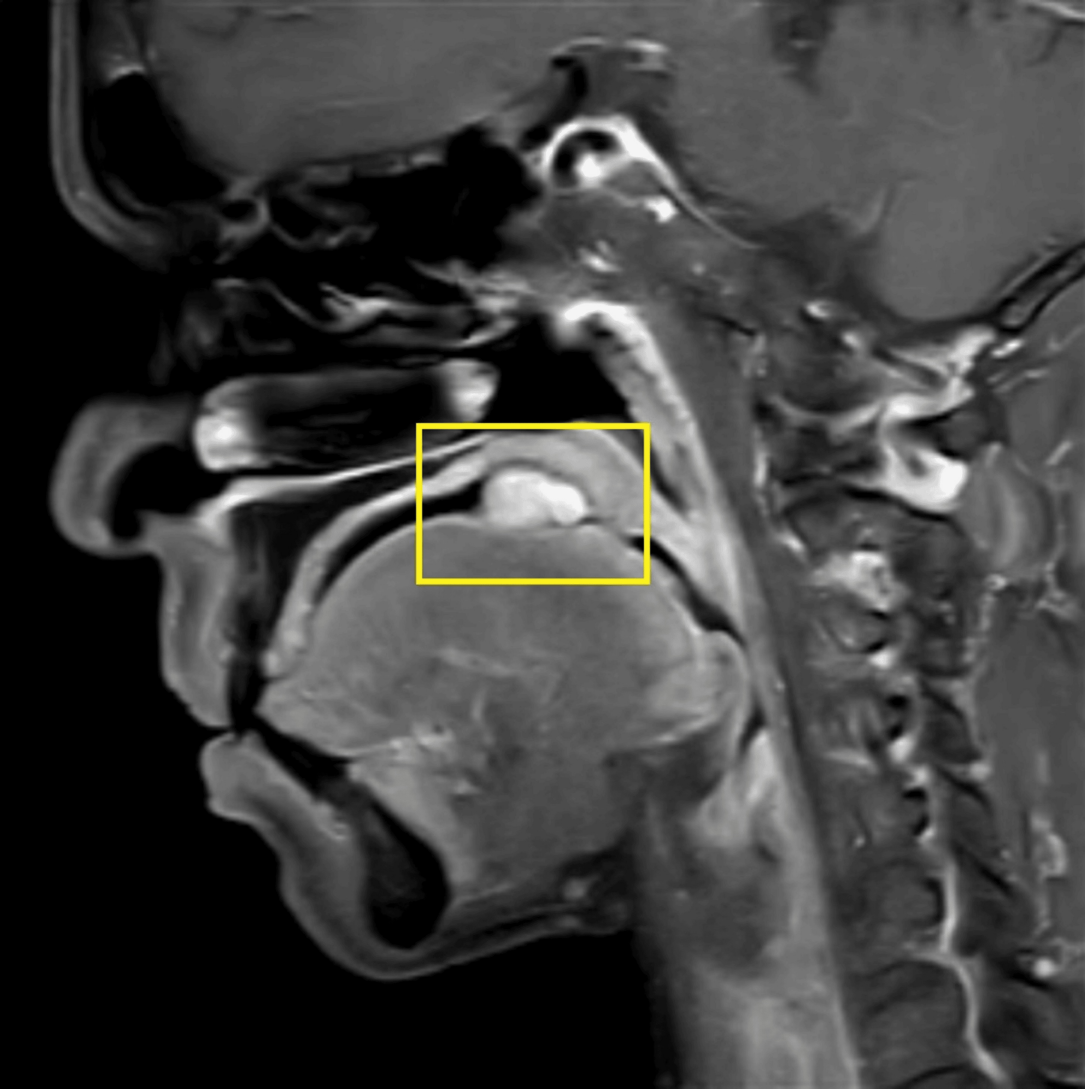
MRI of the oral cavity (sagittal view) MRI: magnetic resonance imaging
